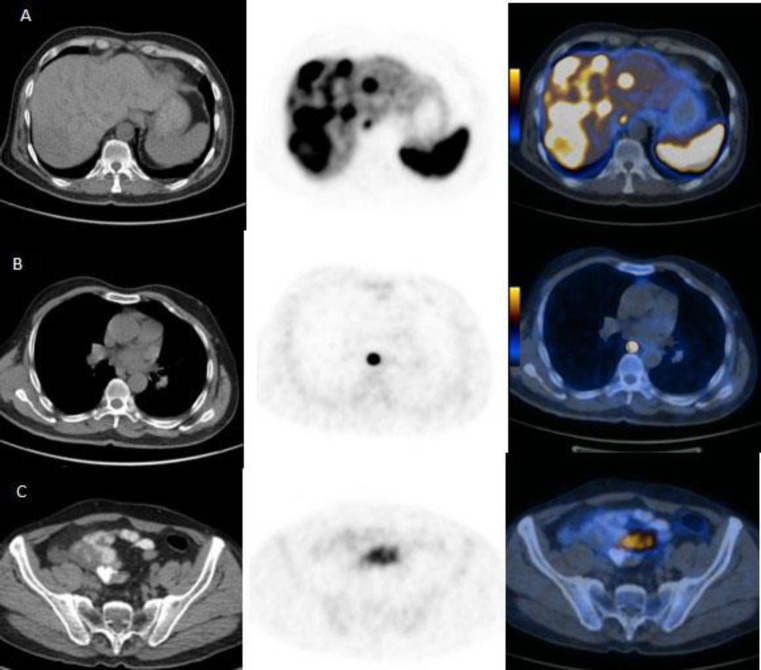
Rows A, B and C shows CT scan, PET and PET-CT image of Liver, Mediastinum and Pelvis involvement by tumor, respectively
